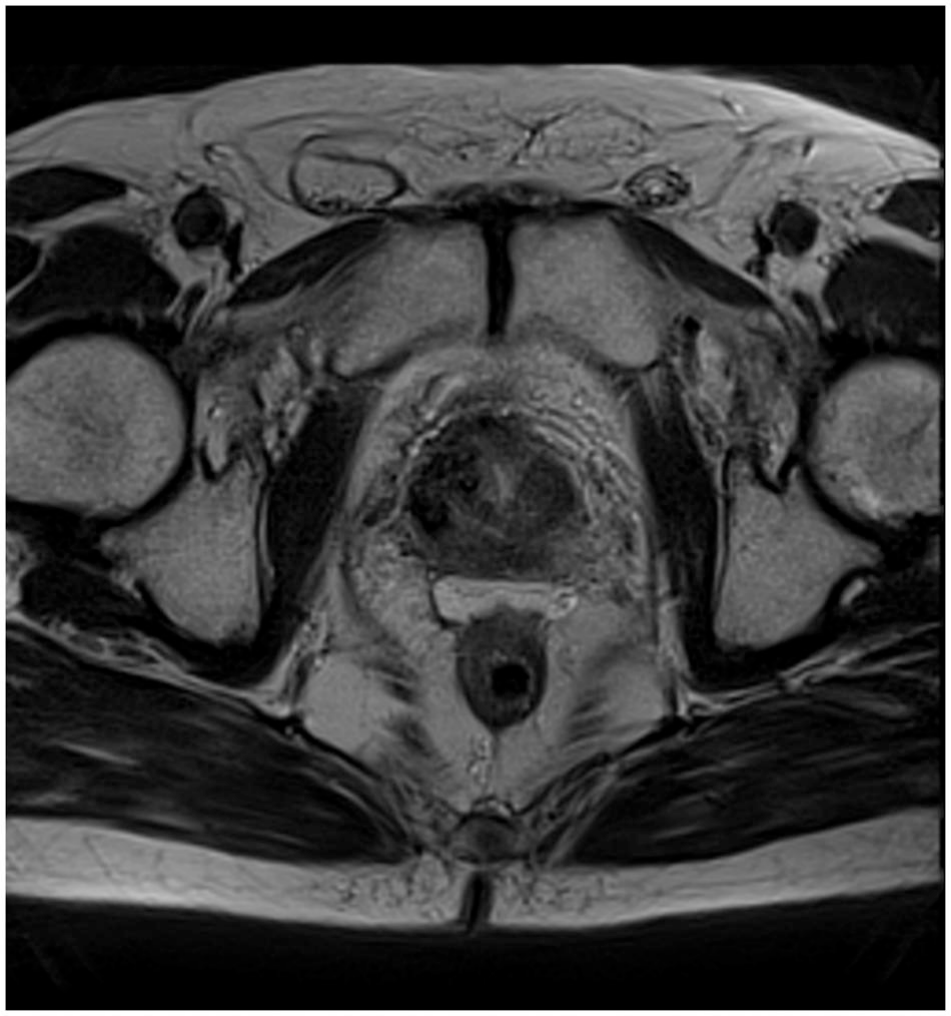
Selected axial MR image of the pelvis 3 months after salvage radiation therapy of recurrent prostate cancer showing anterior rectal wall thickening without fistula formation. The hydrogel SpaceOAR is in an optimal position between the prostate and the rectum, with symmetric distribution.
